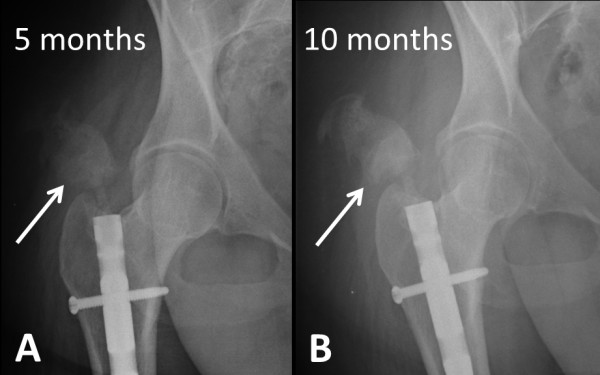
Progressive extent of heterotopic ossification around the right hip is seen tracking down to the entry point of the femoral nail on follow-up X-rays at five months (arrow in A) and 10 months (arrow in B).
